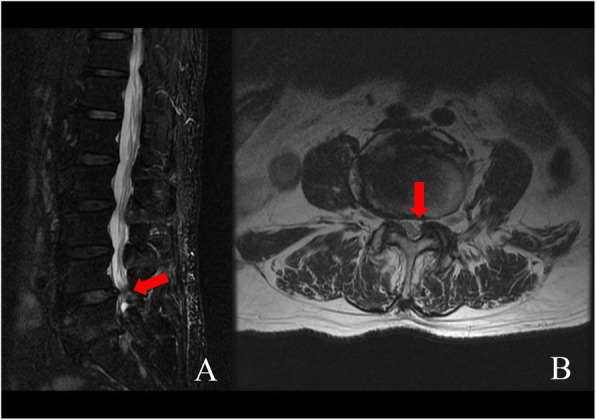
A patient diagnosed with L4/5 spinal stenosis on MRI; (a) Sagittal view demonstrates spinal stenosis at the L4/L5 level (b) Transverse views of spinal stenosis and lateral recess stenosis
